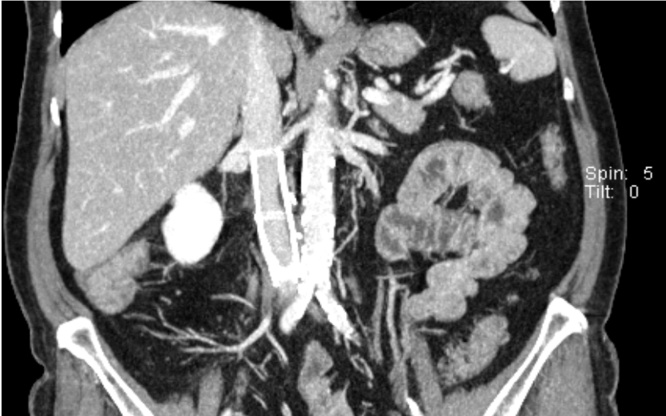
CT evaluation showing no sign of local/systemic disease recurrence 24 months after operation.
